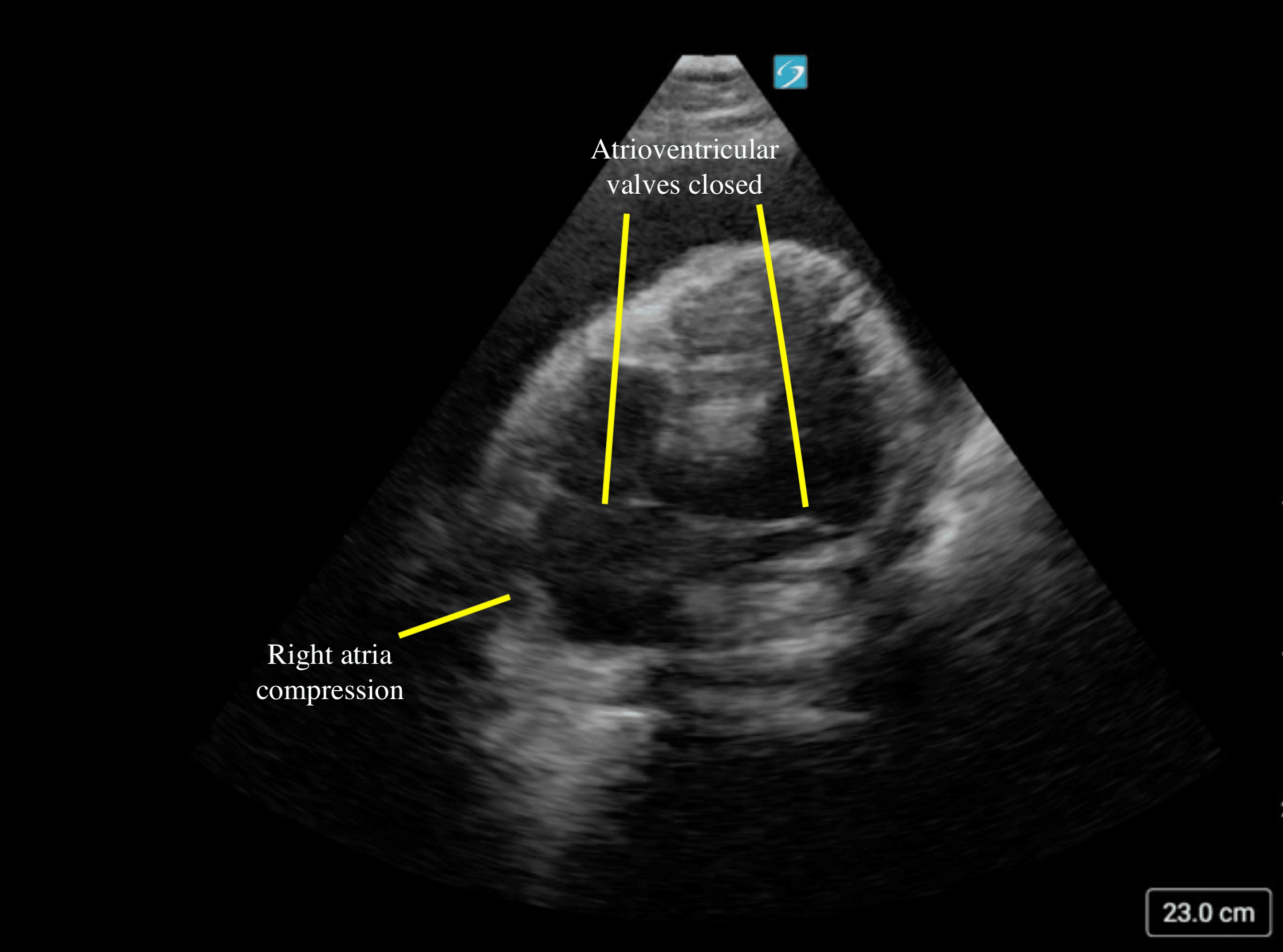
Apical four-chamber tamponade with right atrial compression In this image, a large circumferential pericardial effusion is again visualized. Compression of the right atrium is also noted to occur while the atrioventricular valves are noted to be closed.
